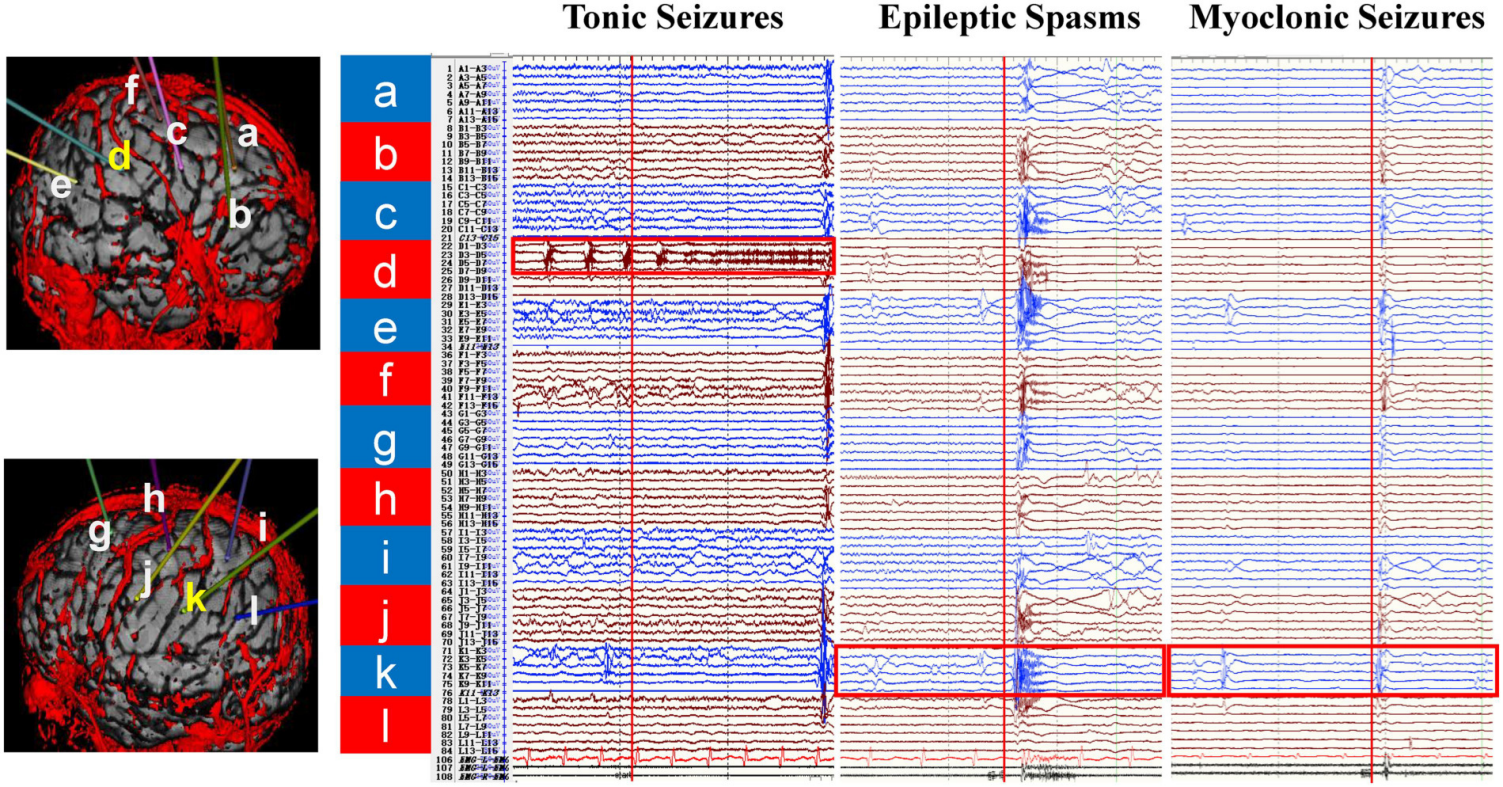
SEEG monitoring results in patients with TSC. Electrodes a-l were implanted in bilateral cerebral hemispheres (a–f) right hemisphere; (g–l) left hemisphere), covering the CT calcified radial lesion resembling TMS and their adjacent cortices. SEEG monitoring showed focal tonic seizures originating from the right lesion (electrode d), and focal epileptic spasms and myoclonic seizures originating from the left lesion (electrode k). SEEG, Stereoelectroencephalogram; TSC, tuberous sclerosis complex; TMS, transmantle sign.
